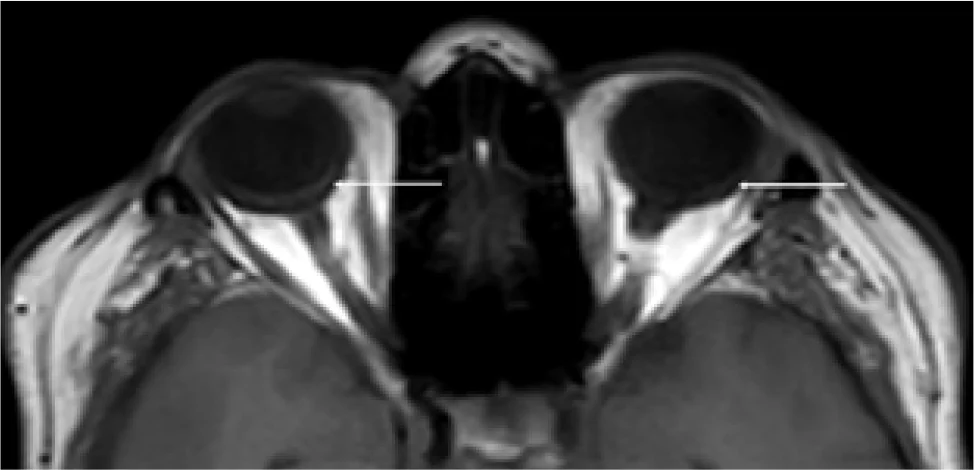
MRI of a 50-year-old woman presenting with headache, bilateral
